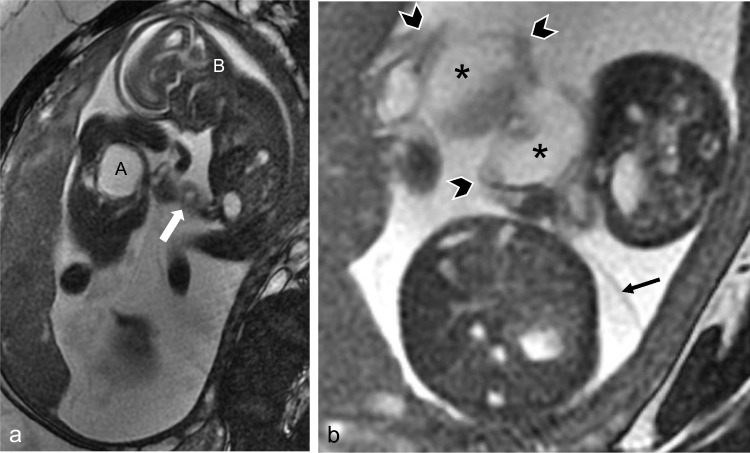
Fetal MRI of diamniotic minimally conjoined omphalopagus at gestational age 19 weeks. a Two-dimensional FIESTA sagittal to twin B shows herniated bowel (*white arrow*) extending across the fused tissue to twin A. b Two-dimensional FIESTA axial to the twins’ separate abdomens shows the umbilical cords (*black arrowheads*) inserting onto the shared allantoic cysts (*black asterisks*). The intertwin membrane of this diamniotic pregnancy is visible (*black arrow*). *FIESTA*, fast imaging employing steady-state acquisition
